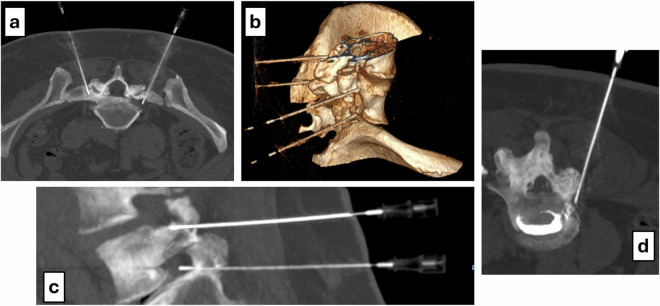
a Axial maximum intensity projection (MIP) showing bilateral L5 foraminal needle placement. b Three-dimensional CT reconstruction showing placement of four needles at the targeted foraminal sites. c Sagittal MIP image demonstrating two needles at the L4 and L5 foramina. d Axial CT showing inadvertent intradiscal contrast spread at the L4–L5 disc level
